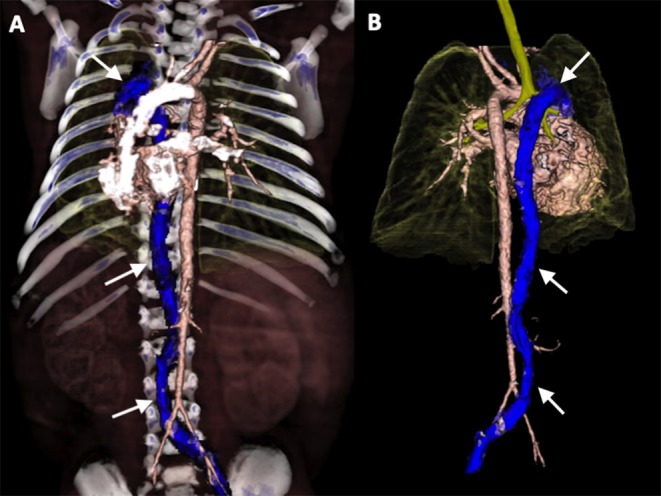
3D-volume rendering reconstructions of Computed Tomography Angiography (CTA) in anterior view (A) and posterior view (B): demonstrating interrupted inferior vena cava with azygos continuation (arrows).
